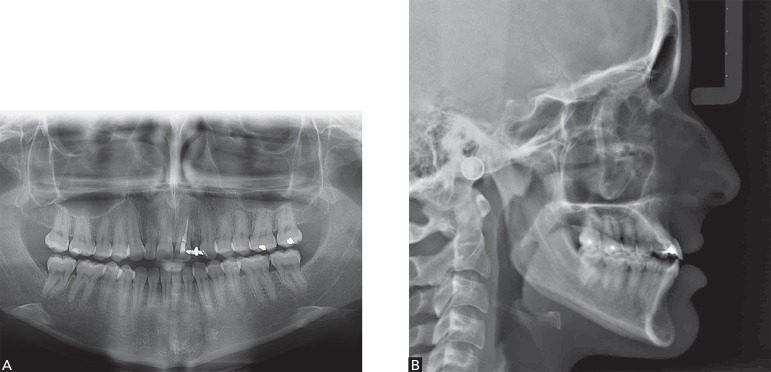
radiographs: A) Panoramic raA B diograph; B) Profile radiograph.
